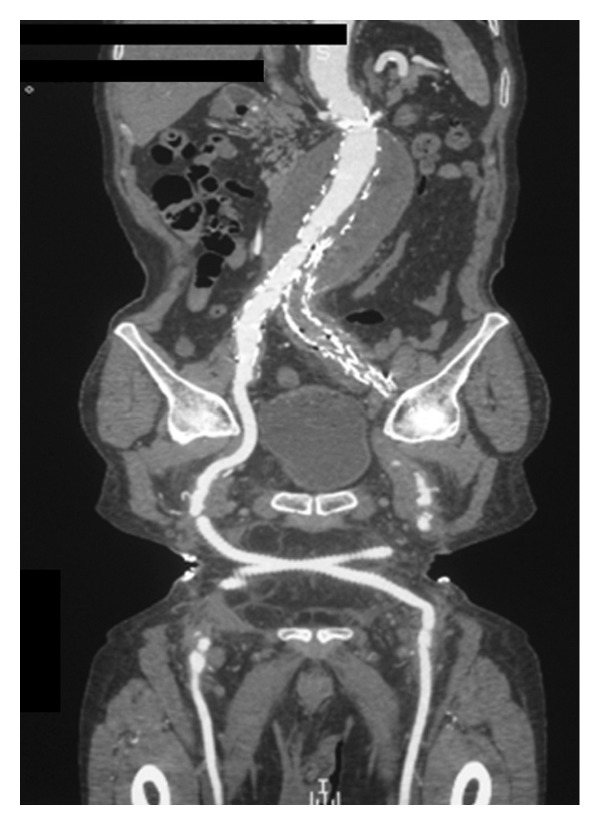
Complete exclusion of the aneurysm on CT angiography one month postoperatively.
